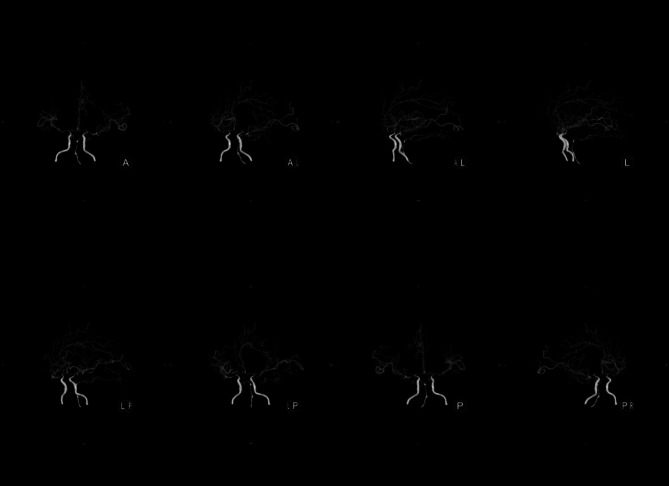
Neck CT angiography scanning
